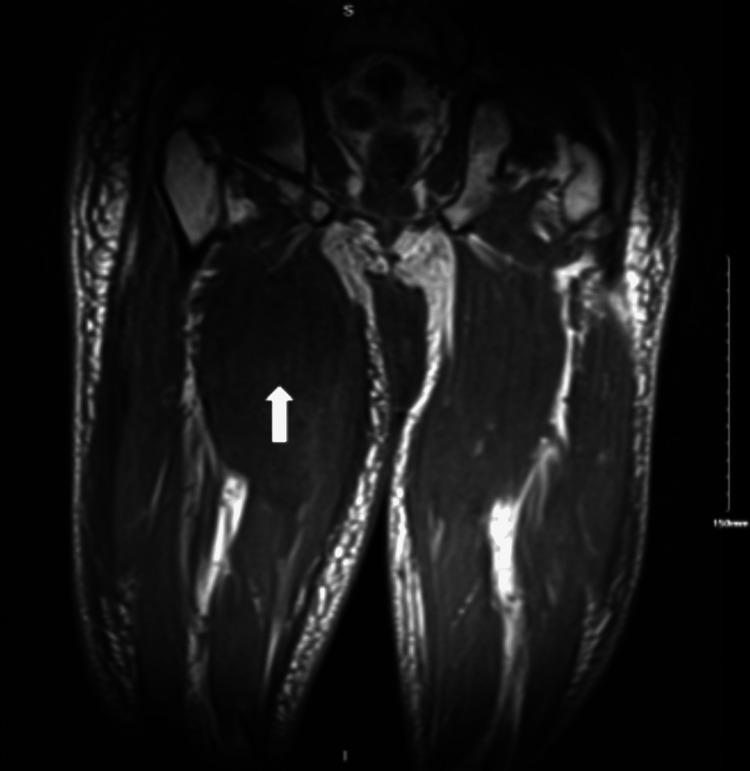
MRI of the right thigh in the coronal section without contrast showing severe myositis with associated superficial and deep fasciitis and the areas of myonecrosis MRI: magnetic resonance imaging
